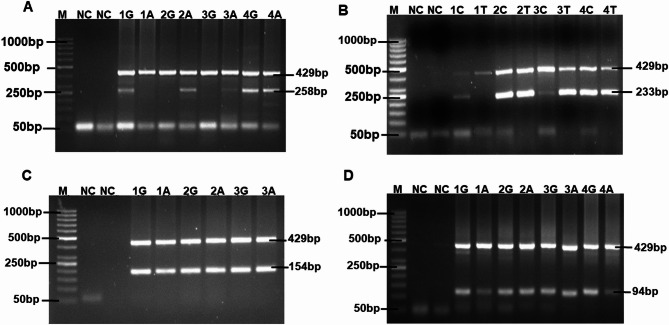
ARMS-PCR of *IL-10* and *TNFα/β* genotypes. Agarose gel electrophoresis of the ARMS PCR assay products. The 429 bp amplification product of the HGH control was present in all lanes, showing optimal amplification. M = 50 bp ladder, NC = Negative control. (A) IL-10 (-1082 > G/A): Lane 1G- 1 A = Homozygous GG; Lanes 2G- 2 A and 3G- 3 A = Homozygous AA and Lane 4G- 4 A = Heterozygous GA for IL-10 (-1082 G > A) polymorphism. (B) IL-10 (-819 C > T): Lane 1 C- 1T = Homozygous CC; and Lanes 2 C- 2T, 3 C- 3T and 4 C- 4T = Heterozygous CT for IL-10 (-819 C > T) polymorphism. (C) TNF-α (308 > G/A) genotypes from ARMS-PCR amplification. From left to right: NC = Negative control; Lanes 1G- 2 A; 3G- 4 A and 5G- 6 A = Heterozygous GA for TNF-α (308 > G/A) polymorphism in sickle cell disease patients. (D) TNF-β (Intron1 + 252 > G/A) genotypes from ARMS-PCR amplification. From left to right: NC = Negative control; Lanes 1G- 2 A; 3G- 4 A and 5G- 6 A = Heterozygous GA for TNF-β (Intron1 + 252 > G/A) polymorphism in sickle cell disease patients
